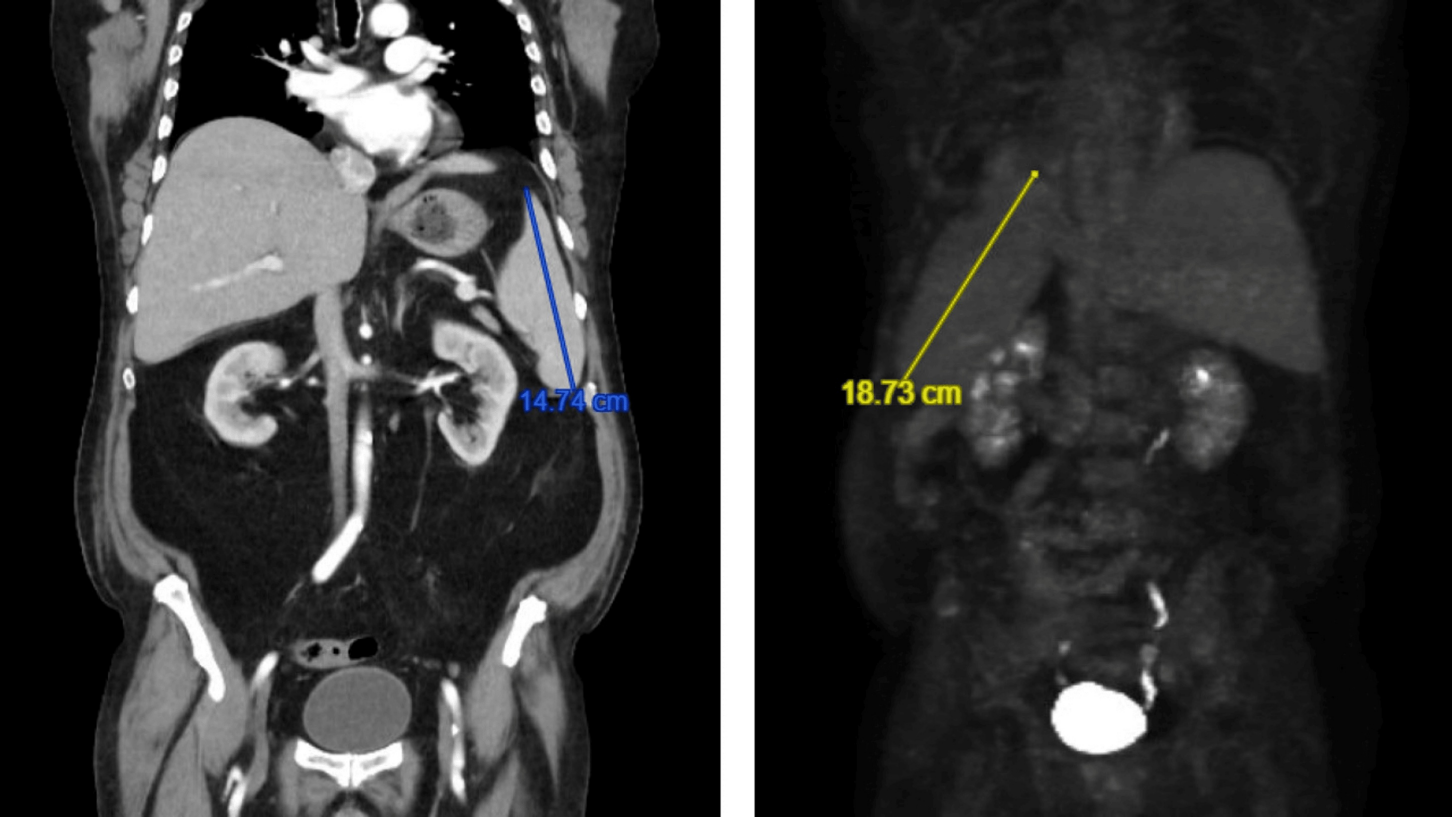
Coronal computed tomography (CT) of the chest, abdomen, and pelvis depicting stable, 14.74 cm splenomegaly (left). Maximal intensity projection from positron emission tomography-computed tomography (PET-CT) depicting worsening, 18.73 cm splenomegaly (right)
